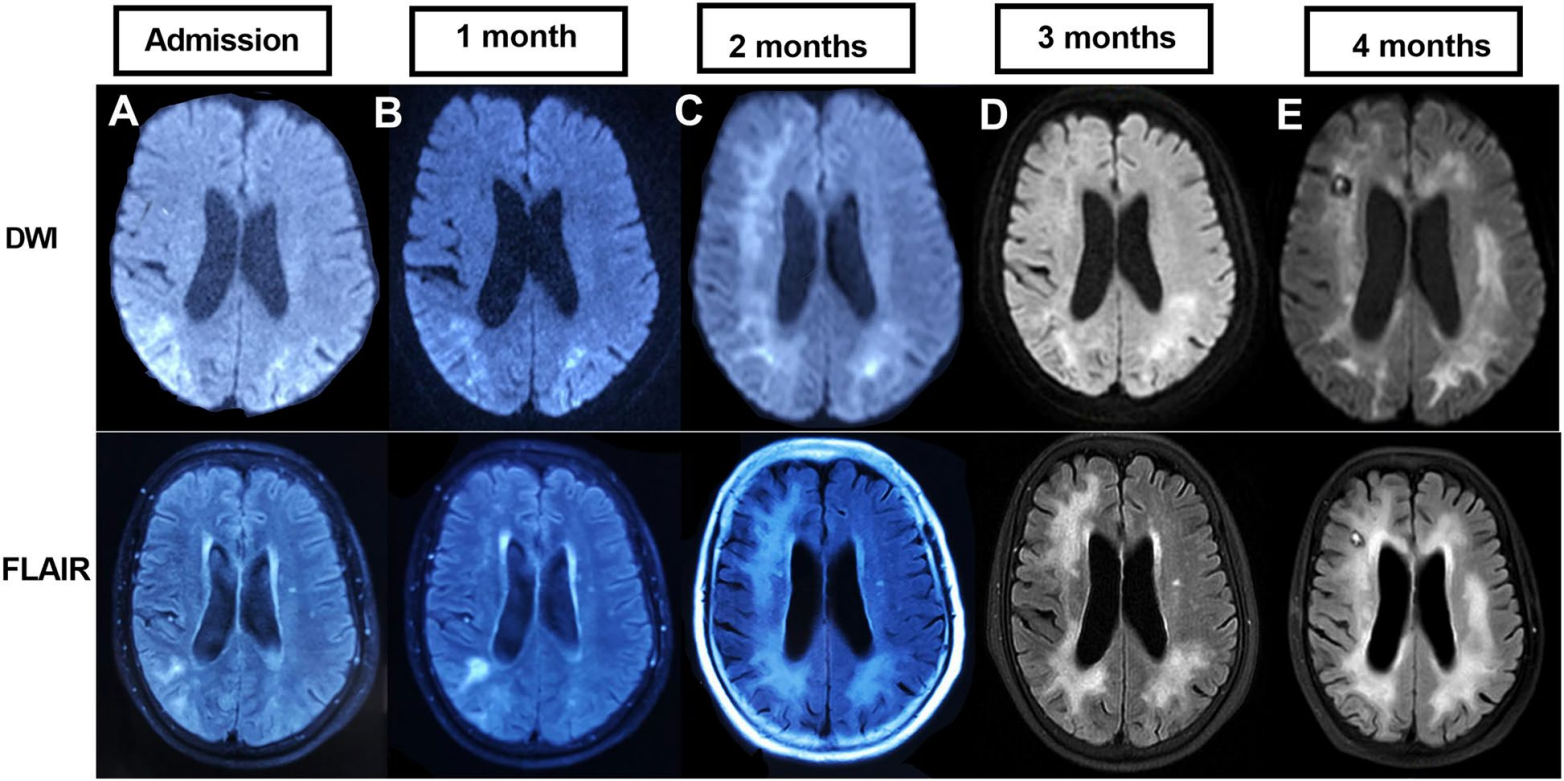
MRI shows hyperintense signal in DWI and FLAIR images. A-E (from the beginning of disease to 5 months) shows that the white matter lesions were gradually aggravated with the progress of the disease
